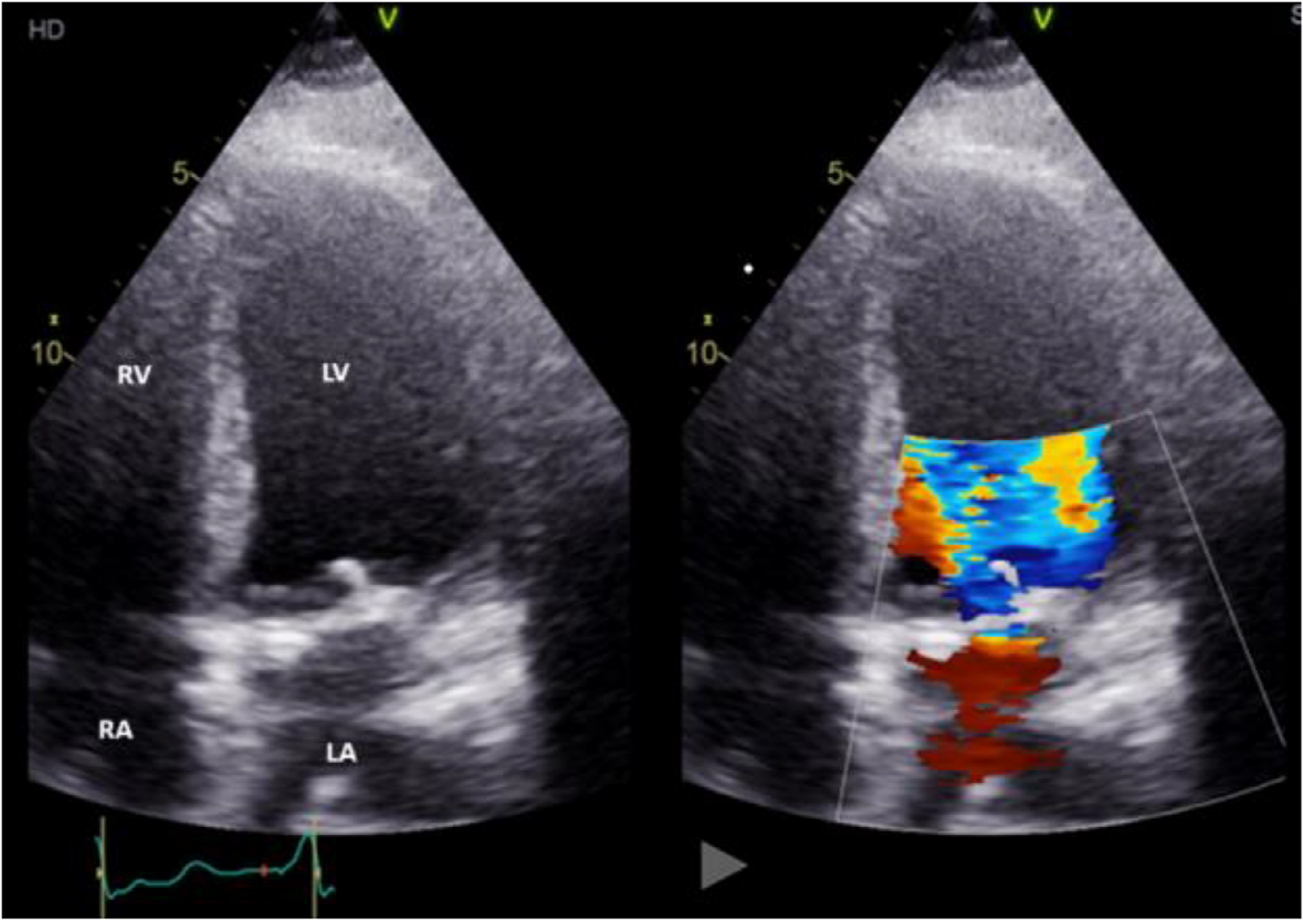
Transthoracic echocardiogram showing severe mitral regurgitation in apical four‐chamber view. The right panel shows color Doppler imaging with turbulent regurgitant flow (blue and orange jets) across the mitral valve into the left atrium. LA, left atrium; LV, left ventricle; RA, right atrium; RV, right ventricle.
